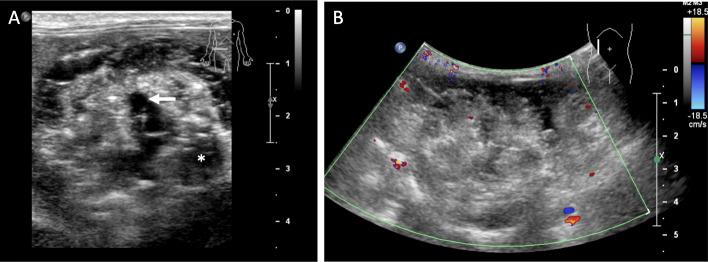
Ultrasound. a Grayscale ultrasound. The teratoma was solid with heterogeneous echogenicity, reflecting the fat content and areas of calcification with acoustic shadowing (asterisk) observed. Anechoic areas were present that may have represented cysts (arrow). b. Color Doppler ultrasound. There was little vascularity on color Doppler imaging
